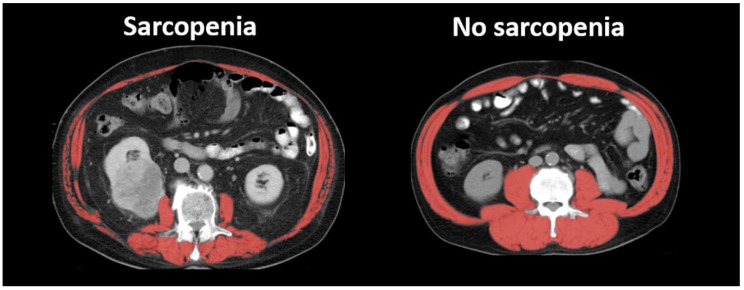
Computed tomography (CT) images of typical sarcopenic and non-sarcopenic cases. Skeletal muscle area is shown in red.
